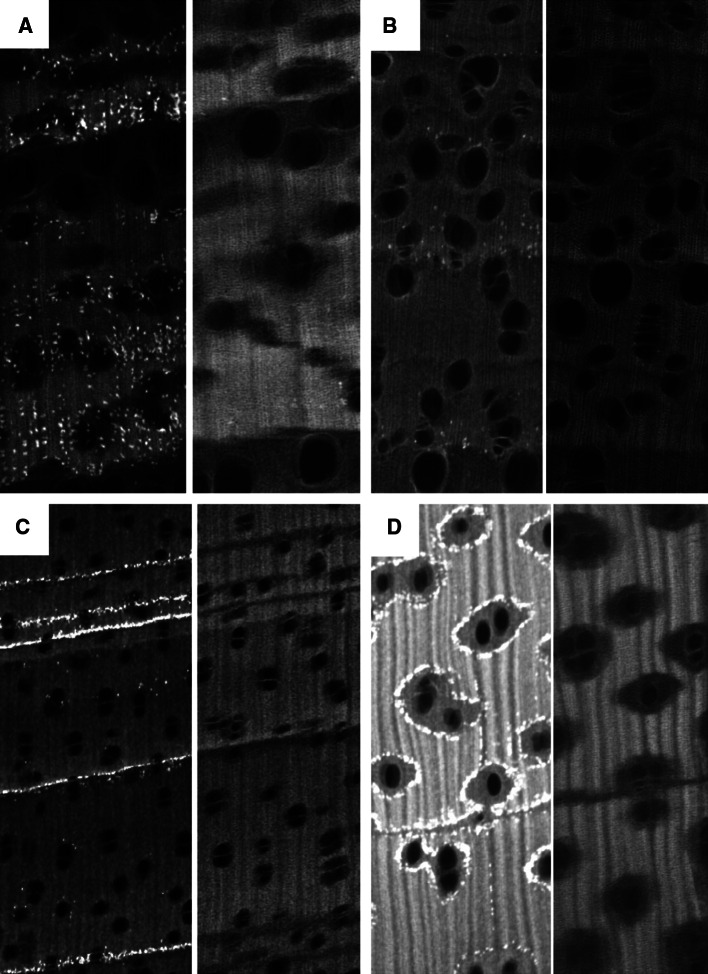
Comparison of X-ray images of samples of untreated wood (*left half of image*) and extracted with HCl (*right half*). a
